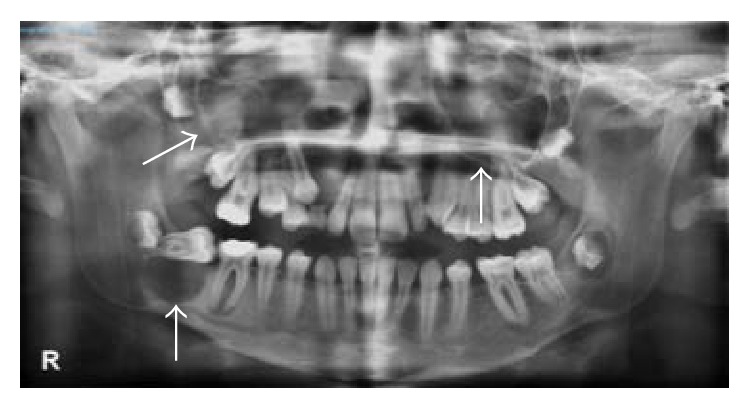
Orthopantomography. Odontogenic cysts (arrows).
